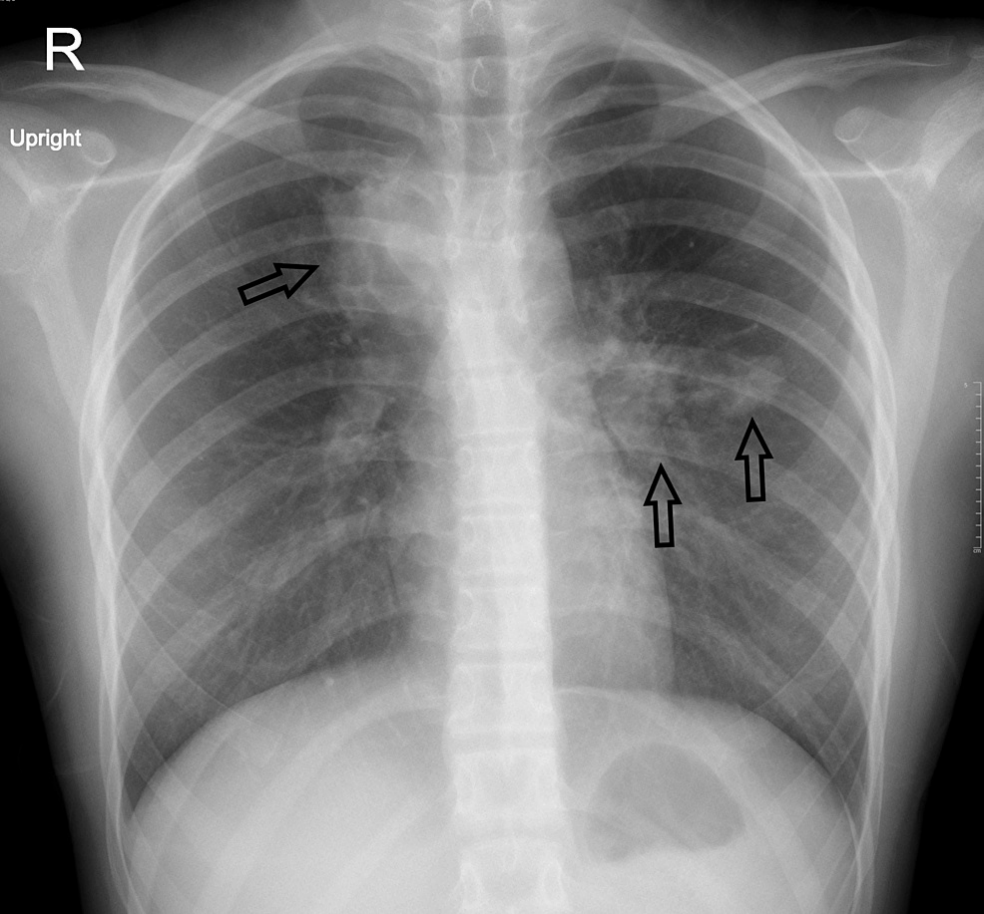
Repeated chest x-ray showing an increase in the mediastinal mass (black arrows).
